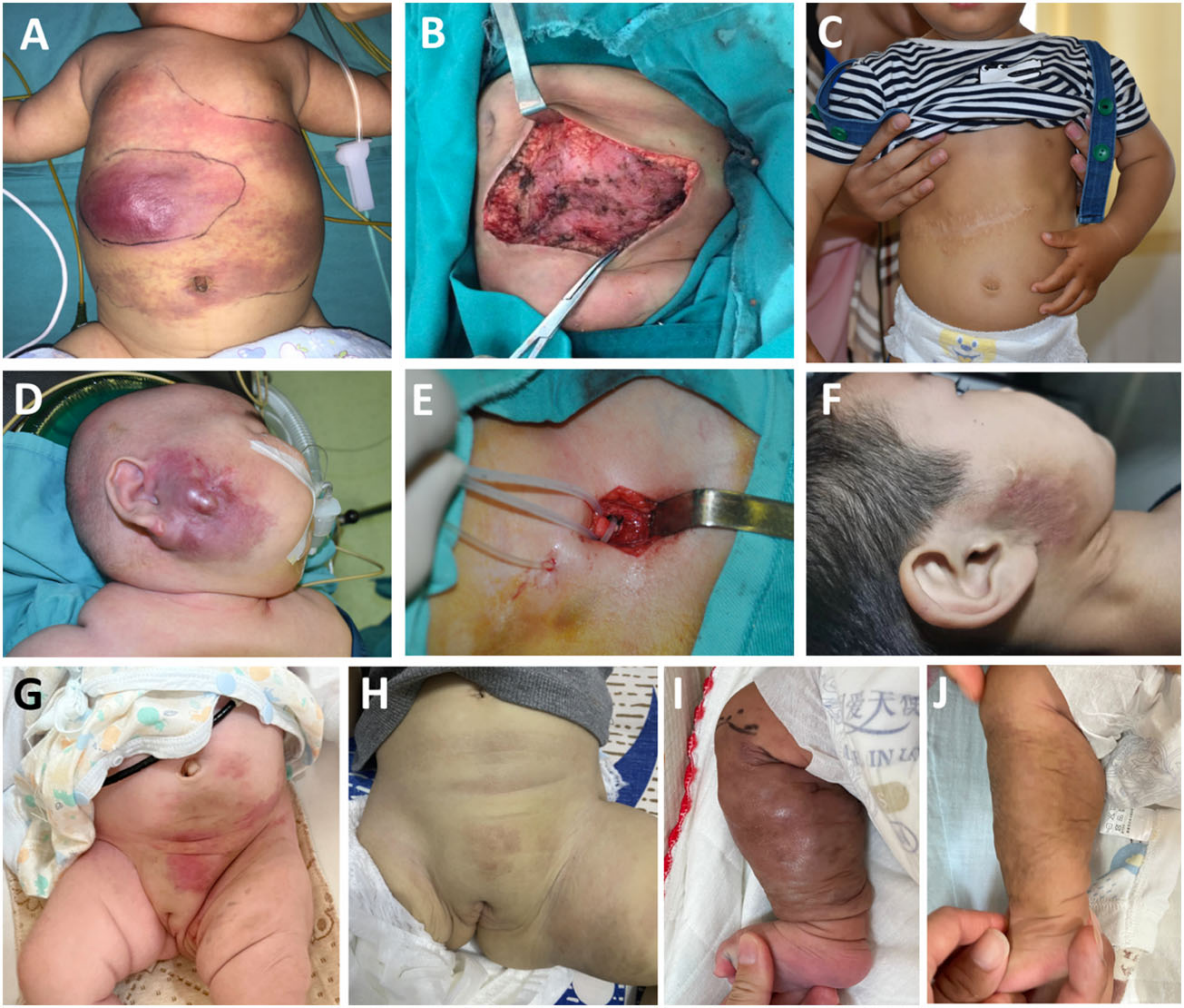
Individualized comprehensive treatment for patients with Kaposiform hemangioendothelioma at different degrees/stages: a 115-day-old boy with enlarging pink mass before operation, after operation, and after 5 years of follow-up (A–C). An 8-month-old boy diagnosed with KMP (D), then with external carotid artery ligation treatment (E); sirolimus was taken afterwards with 5 years of follow-up (F). A 2-day-old girl associated with thrombocytopenia and consumptive coagulopathy (G); methylprednisolone and sirolimus were used with 1-year follow-up (H). A one-day-old boy diagnosed with KMP with a lesion invading almost the entire circumference of the right calf (I). Sirolimus was taken after 1-year follow-up (J). Related MRI scans before and after treatment can be found in supplementary documents.
